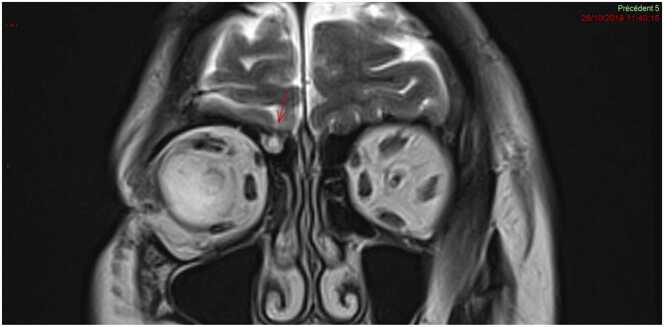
Imaging prior to the CSF leak. Cerebral MRI (T2 sequence) from 2019 in the coronal plane demonstrating a meningocele (arrowhead) situated under the right ethmoid prior to nasopharyngeal testing for COVID-19. COVID-19, coronavirus disease 2019; CSF, cerebrospinal fluid; MRI, magnetic resonance imaging.
